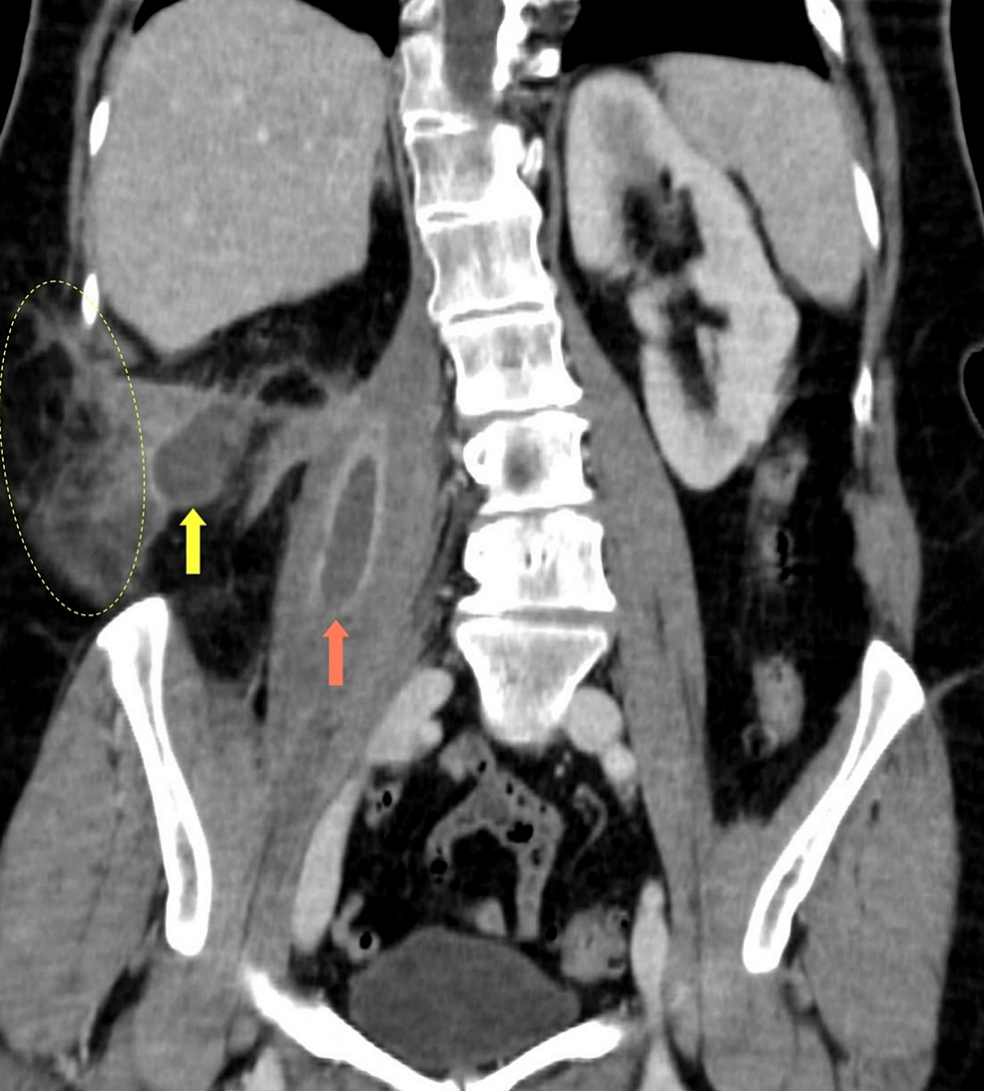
Coronal section of the abdominal CT scan performed on admission, showing abscesses in the psoas (orange arrow) and quadratus lumborum (yellow arrow) muscles, and local cellulitis adjacent to the latter (ellipse).
